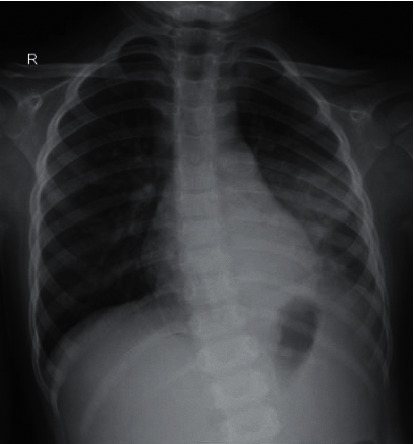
AP-supine chest radiograph: visible consolidation in the left inferior lobe of the lungs. The right lung had no apparent abnormality.
